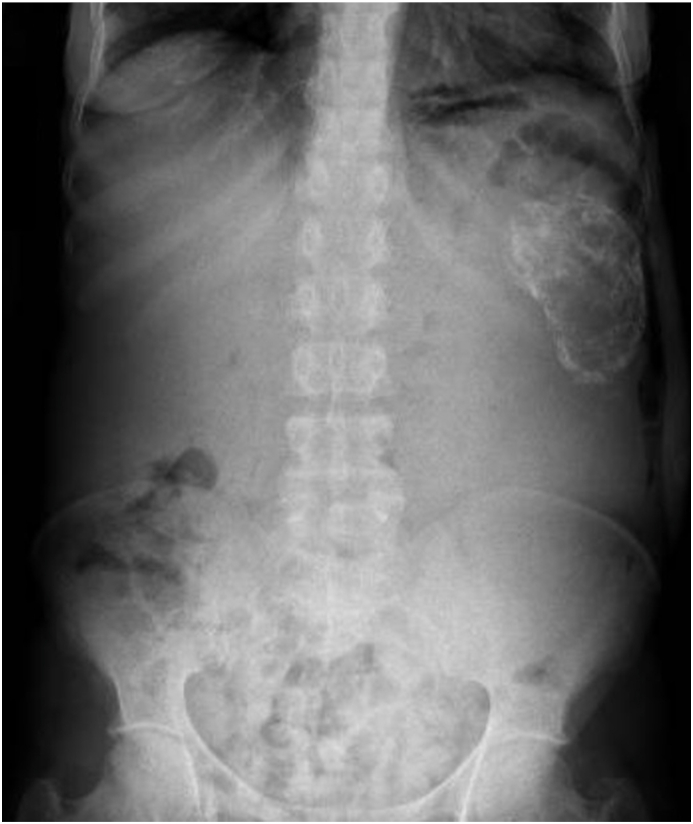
Abdominal X-ray showing a calcified lesion in the left upper quadrant.
